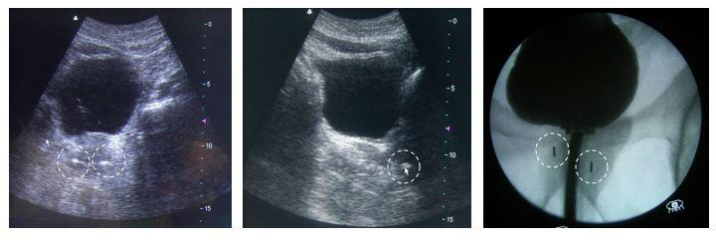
US and x-ray images of the CyberMark™ markers (dotted circles) in the brachytherapy patient.
